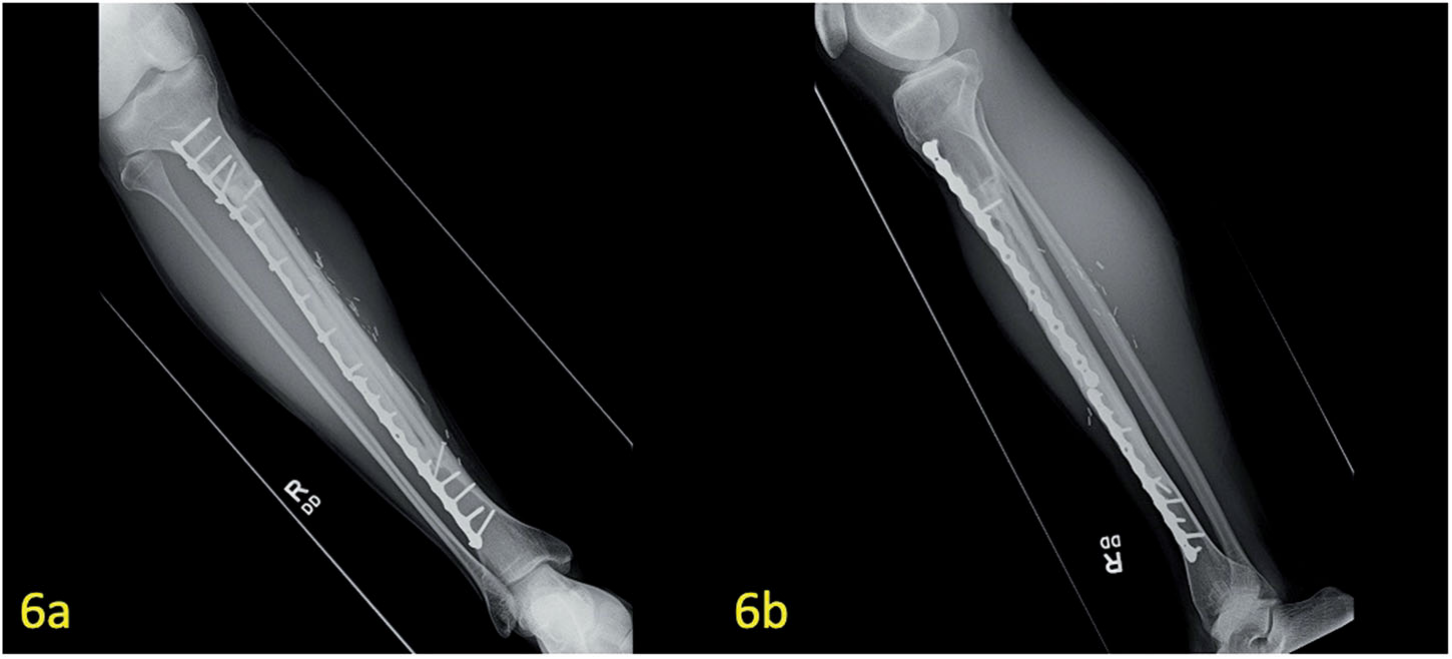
(a,b) Post-operative X-ray of free microvascular fibular graft at 7 years postoperatively. Union of the hemi-tibia allograft and free microvascular fibular graft with proper alignment of hardware can be seen on the AP/lateral radiographs.
